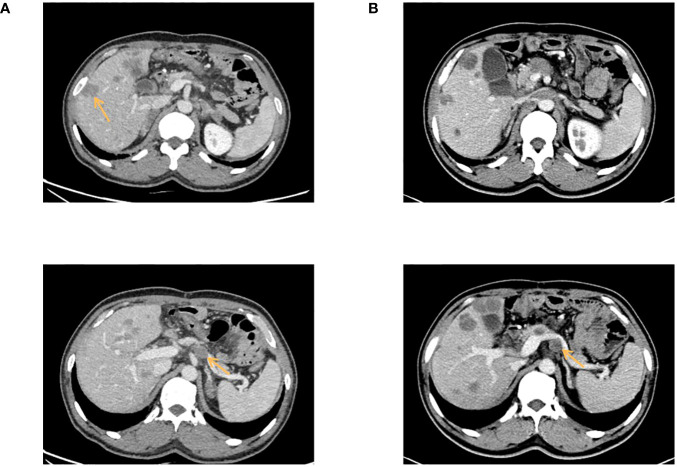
(A) Enhanced CT showed tha the liver had new metastasis. (B) Enhanced CT showed the maximum short diameter of abdominal and retroperitoneal lymph nodes was increased, and the maximum long diameter of intrahepatic metastasis was larged. The arrows indicate the locations of new metastases in the liver and the locations of enlarged retroperitoneal lymph nodes.
